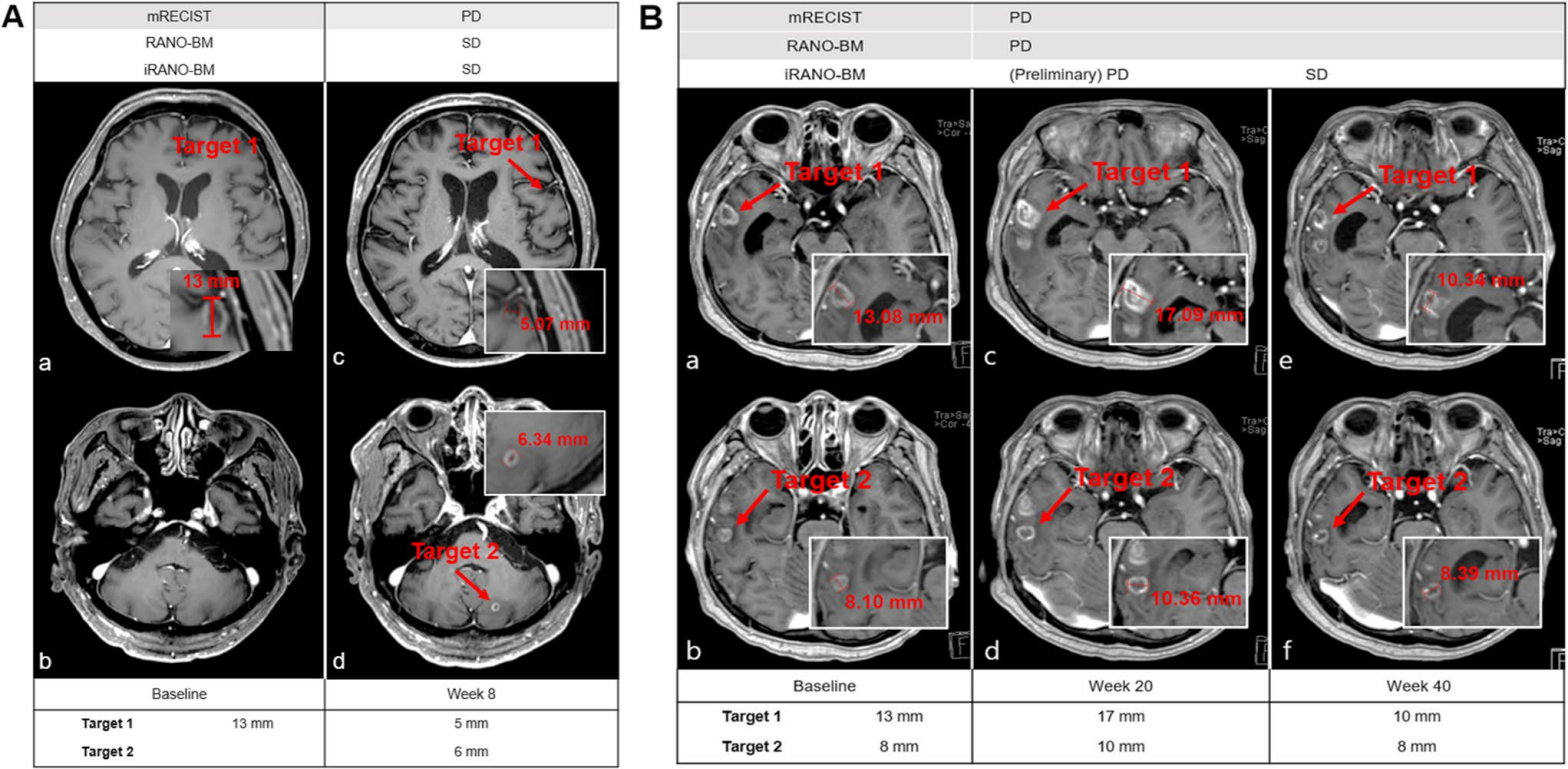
Baseline and Follow-up MRI Scans of a Patient with Different Response Assessments A. Baseline scan (a, b) shows a target lesion of 13 mm. First follow-up scan at week 8 (c, d) shows that the target lesion has decreased in size from 13 mm to 5 mm, and that a new lesion of 6 mm has appeared. Since a new lesion has developed, progressive disease (PD) is defined by mRECIST. However, according to the RANO-BM and iRANO-BM criteria, the diameter of the new lesion is added to the sum of the longest diameter (SLD), which makes the SLD 11 mm for the follow-up scan. Therefore, the response assessment would be stable disease (SD) by RANO-BM and iRANO-BM. B. Baseline scan (a, b) shows two target lesions with sizes of 13 and 8 mm. On the first follow-up scan at week 20 (c, d), the size of each target lesion has increased, and the response assessment would be progressive disease (PD) by mRECIST and RANO-BM. However, this MRI was taken within 6 months of immunotherapy-treatment initiation, and follow-up imaging after 3 months is required for iRANO-BM assessment. In the follow-up MRI at week 40 (e, f), the size of the target lesions has decreased, and this patient is diagnosed with stable disease (SD) by iRANO-BM
